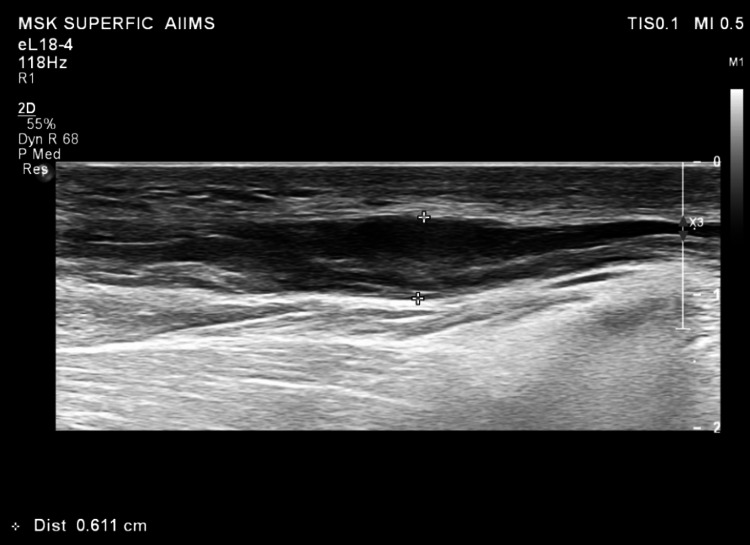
Longitudinal ultrasound view of swelling over the radial cutaneous nerve showing an avascular, heterogeneous hypoechoic lesion over a focally thickened nerve with loss of the normal fibrillar pattern
